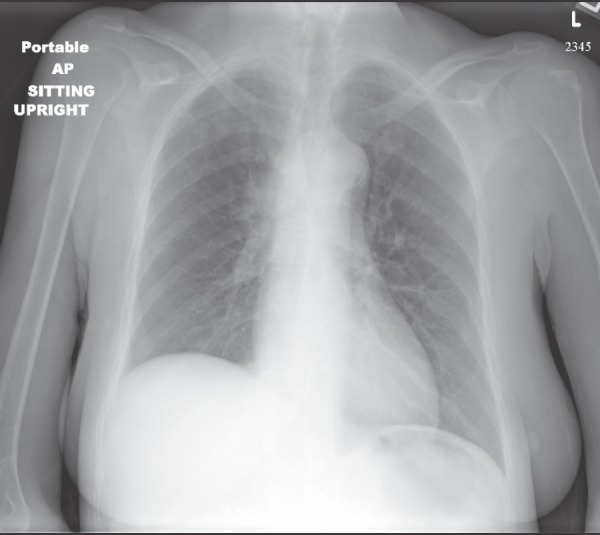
Chest x-ray demonstrating an elevated right hemi-diaphragm, mediastinal lymphadenopathy, and tracheal compression near the level of the carina.
